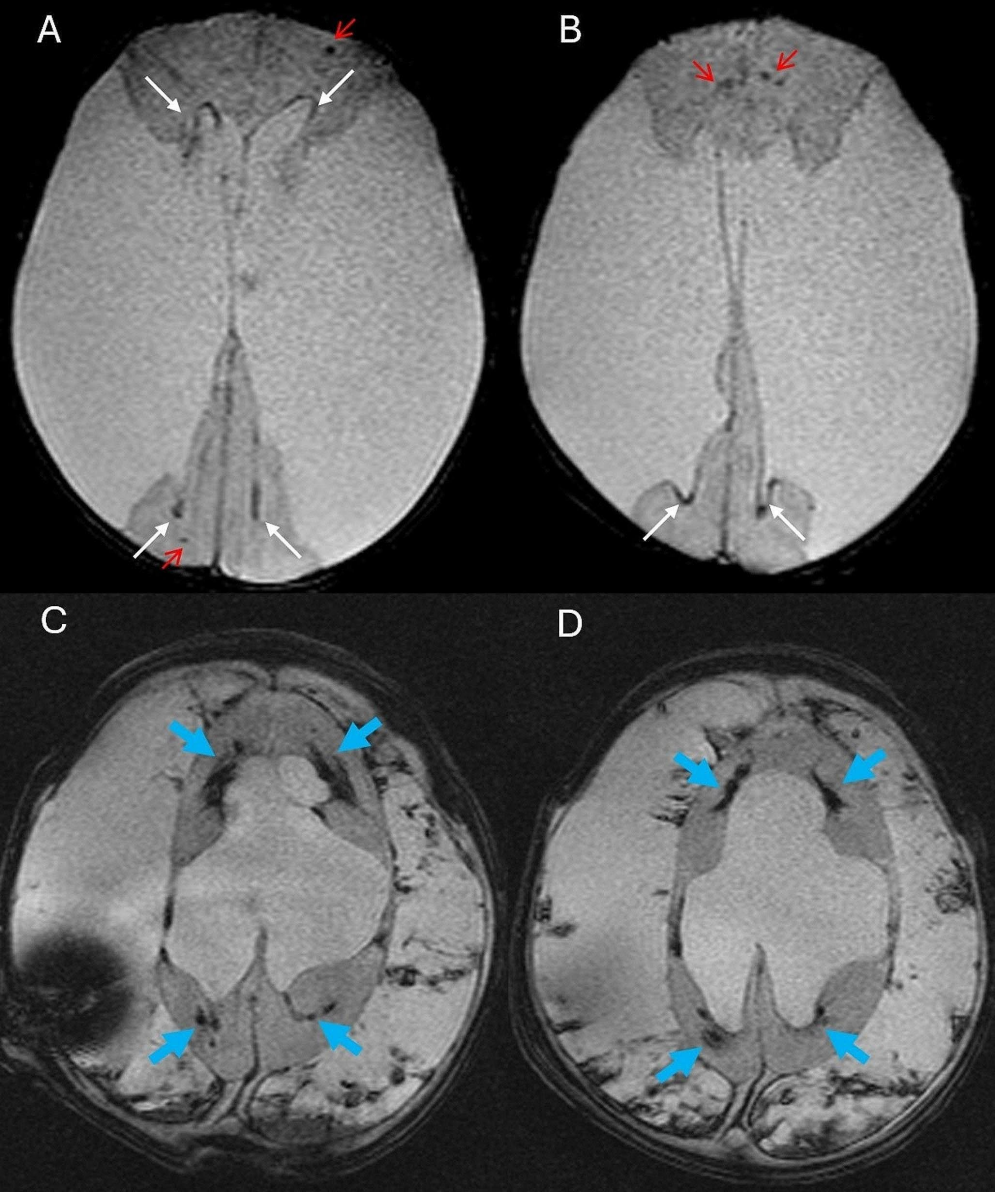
(a-b) Axial T2* imaging pre-shunting in a 3 day old with a COL4A1 mutation demonstrating post-haemorrhagic hydrocephalus in communication with bilateral large porencephalic cysts. Some areas of susceptibility are seen along the ependymal margins of the ventricles (white arrows) and in the frontal and occipital white matter (red arrows), which are shown to represent calcifications on CT (see Fig. 5), a finding related to the underlying COL4A1 mutation. (c-d) Axial T2* MRI at 3 months of age 13 days after insertion of a right transparietal VP shunt showing extensive confluent bilateral fan-shaped periventricular and deep white matter WHIPS (blue arrows) similar to the “iris sign” in deep medullary vein haemorrhage, these are most prominent in the frontal and parietal regions. There are also large bilateral post-operative haemorrhagic subdural collections with worsened hydrocephalus
